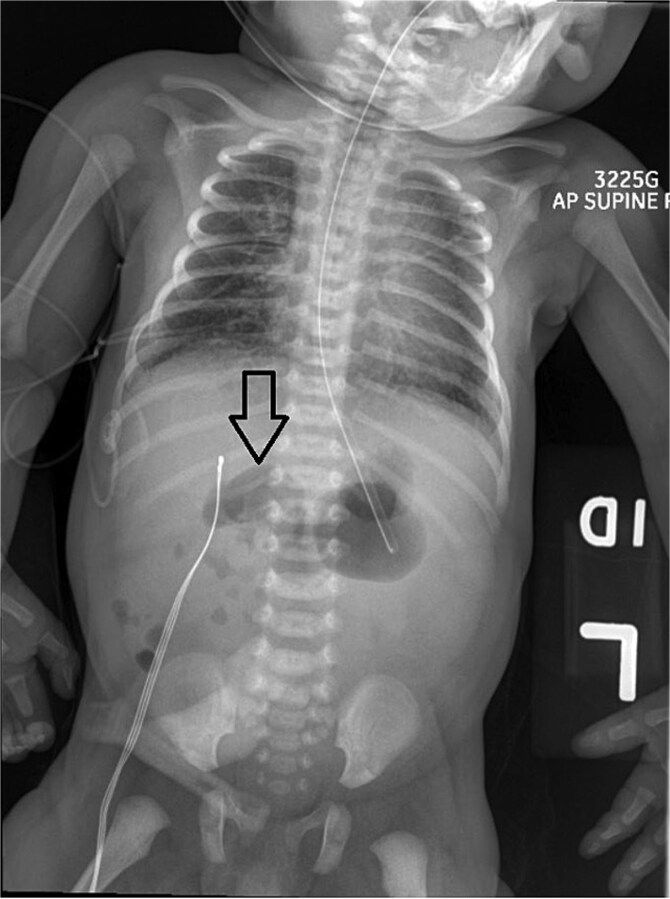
Abdominal X-ray shows nonspecific gas pattern with gas-filled, nondistended bowel loops on the right. Absence of small bowel gas on the left raises suspicion for malrotation.
